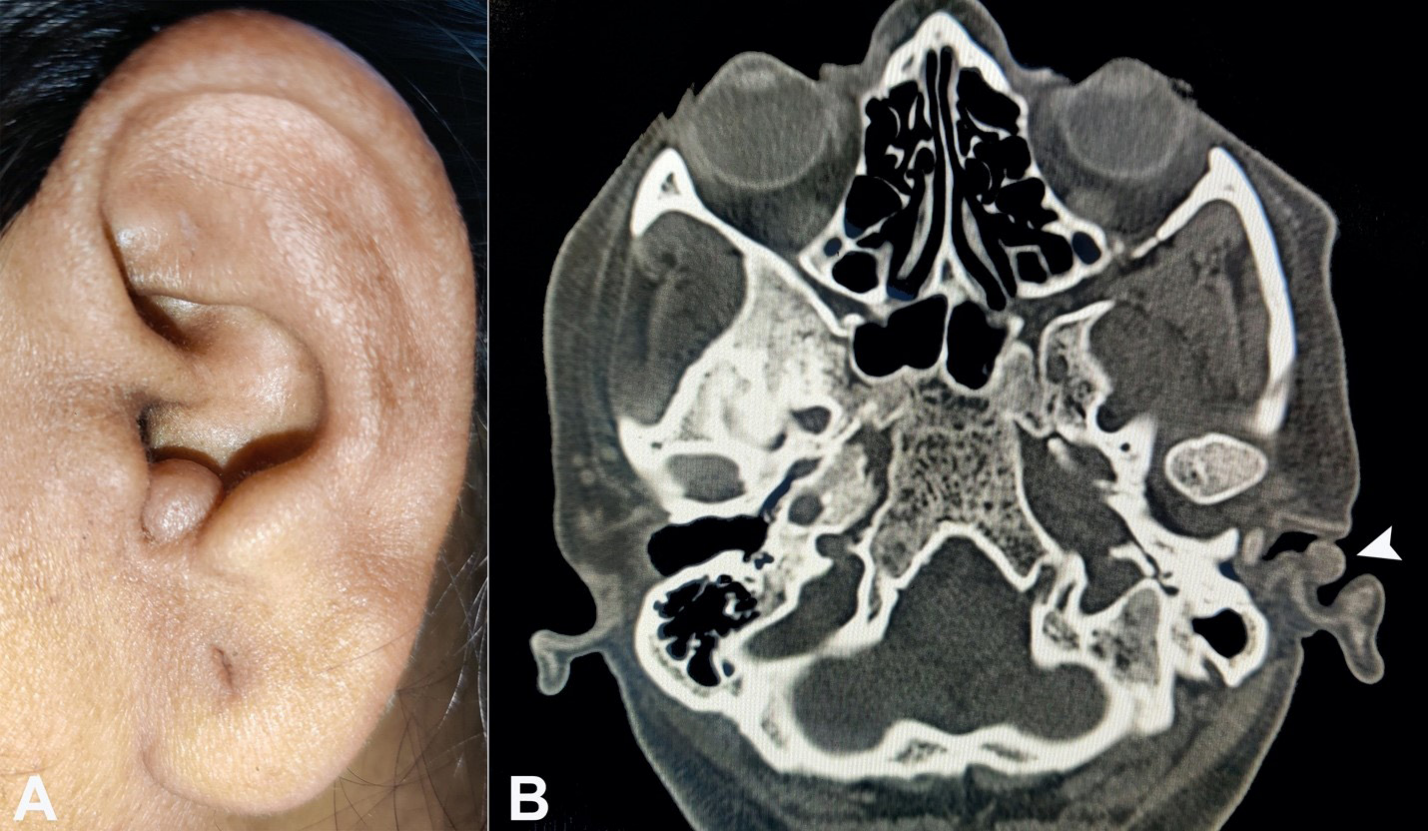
A - gross view of the lesion over the left ear’s cavum concha; B - head computed tomography, axial view showing a homogeneous, well-circumscribed, round lesion over the lateral aspect of the left external auditory canal and adjacent to cavum concha (arrowhead).
